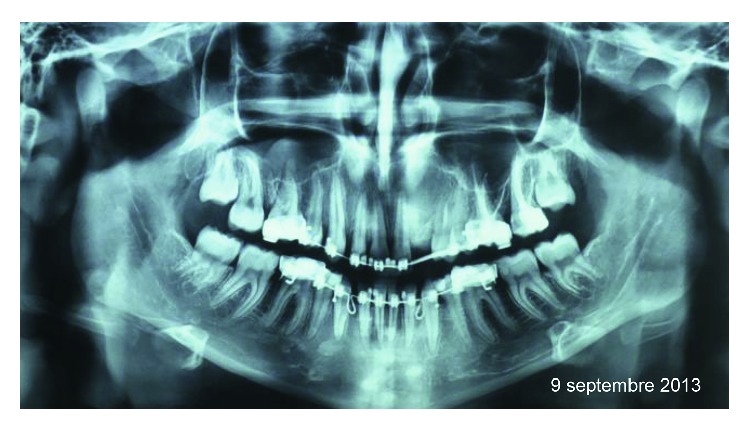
Orthopantomogram 3 months after surgery.
